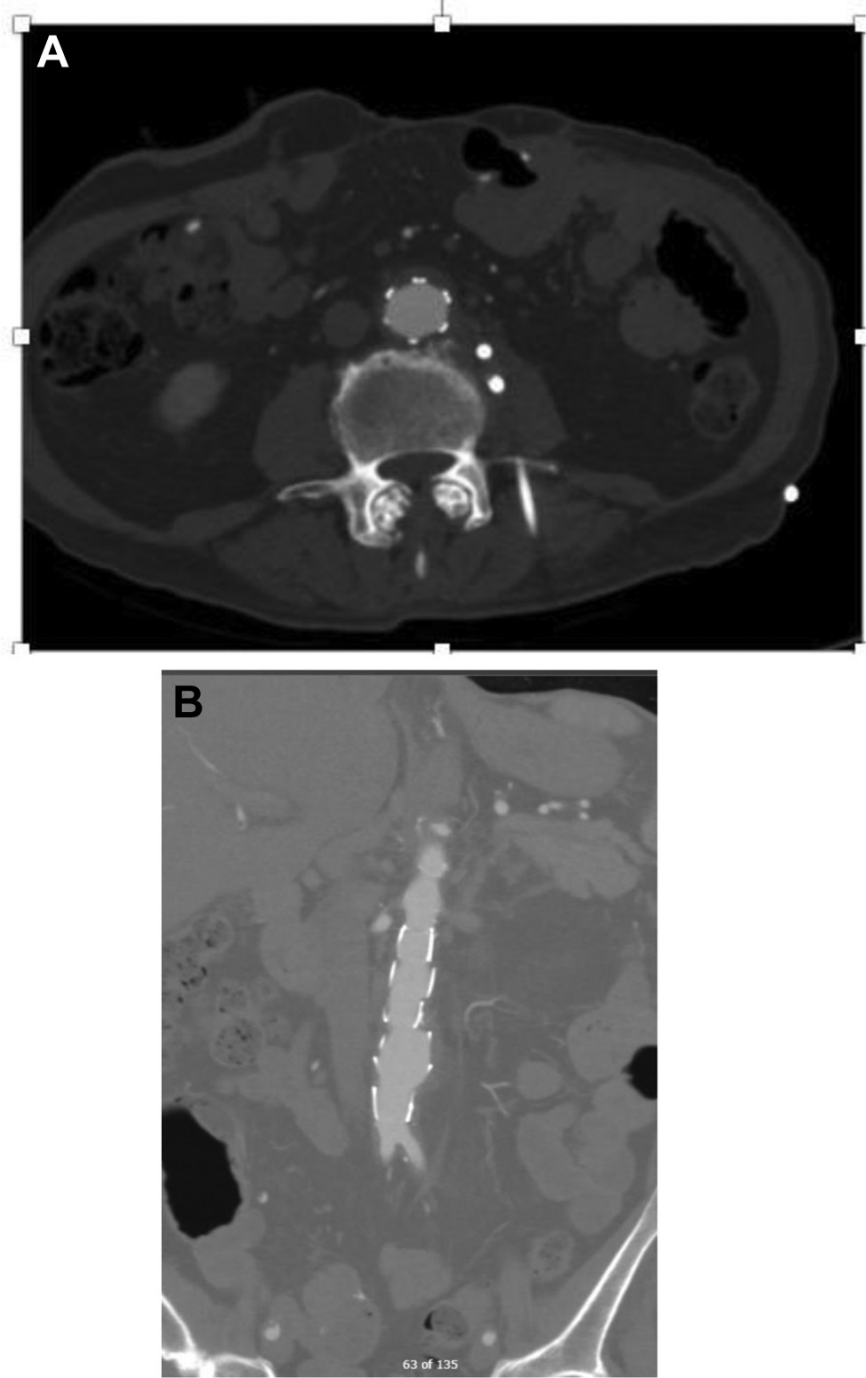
A, Postoperative computed tomography (CT) scan with good apposition of the endograft and associated interventional radiology–placed retroperitoneal drain. B, Follow-up imaging at 6 months with no radiographic evidence of recurrence.
